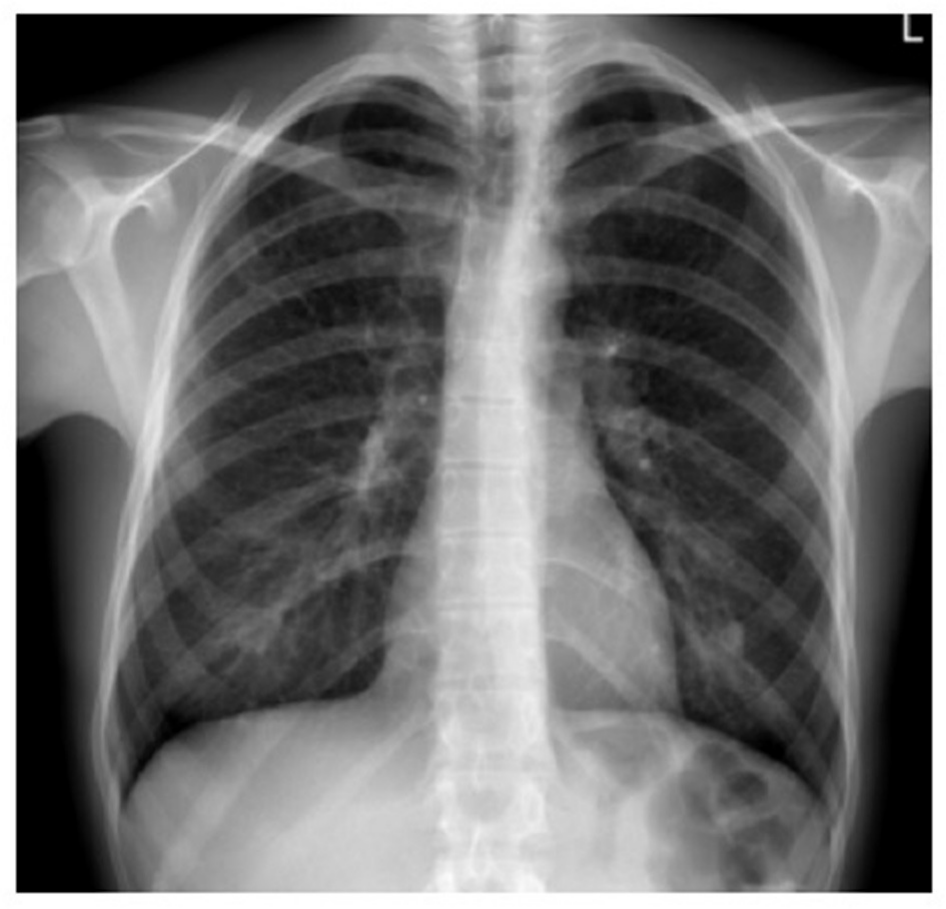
X-ray film of a pulmonary Langerhans cell histiocytosis (PLCH) patient. Multiple reticular and nodular changes in the upper and middle parts of both lungs, with an increase in lung volume.
